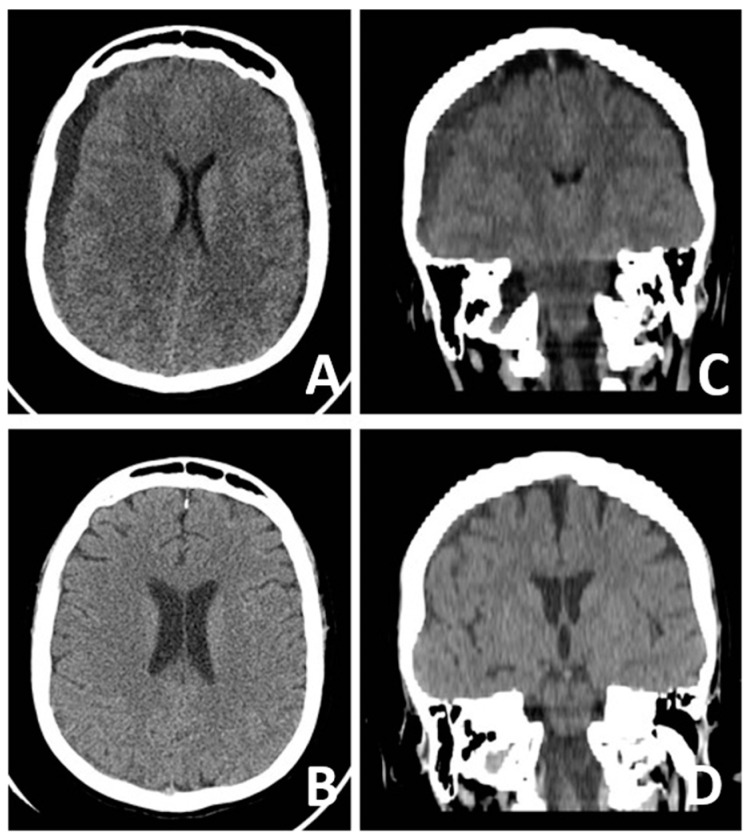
(A,C): Axial and coronal head CT scan images, acquired at admission, showing bilateral hemispheric subdural collections, the thickest of which are on the right, with minimum mass effect. (B,D): Axial and coronal head CT scan images, acquired one month after the patient was discharged, showing the almost complete resolution of the bilateral hemispheric subdural collections.
